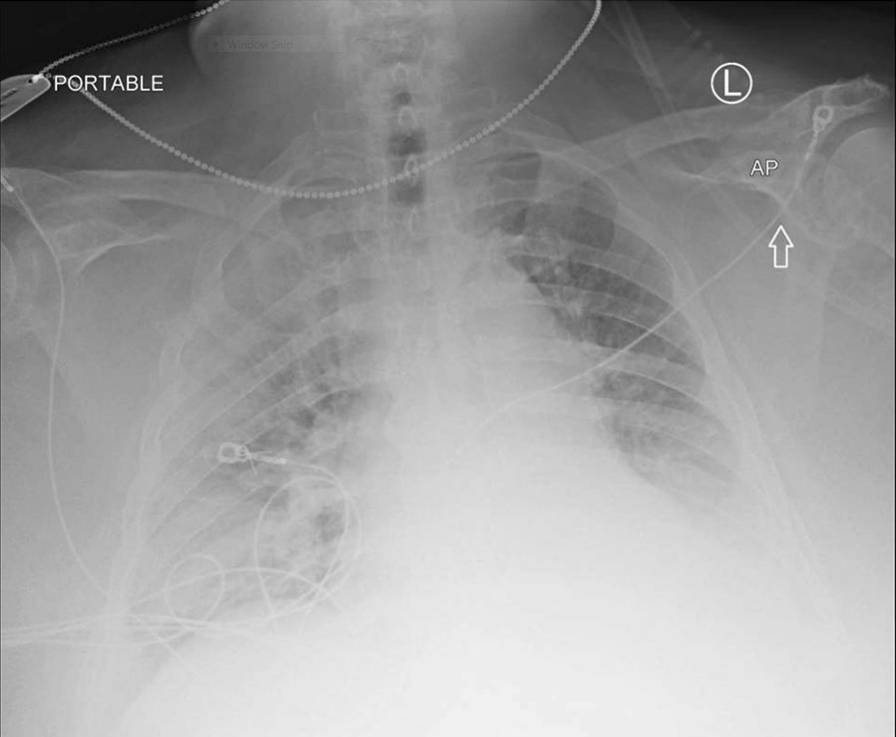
Chest X-Ray in June 2020 with coronavirus disease 2019 reinfection
